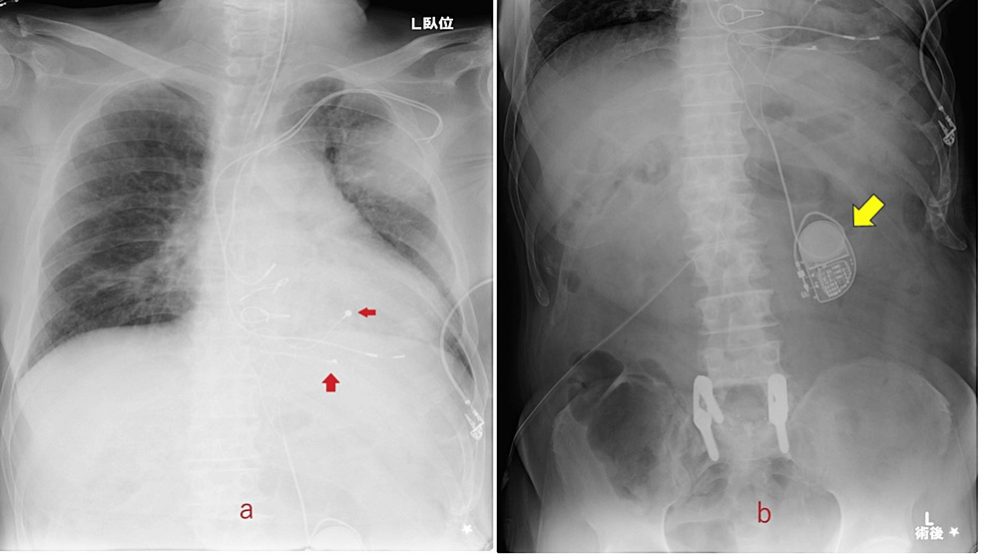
Chest and abdominal X-ray films. Arrows show the electrodes of the new lead attached to the epicardium. The new pacemaker (yellow arrow) implanted in the abdomen.
